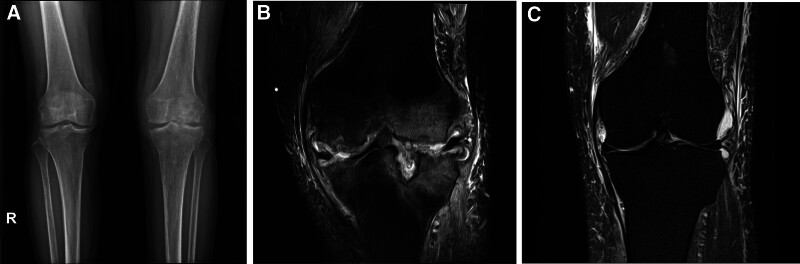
Radiography and magnetic resonance imaging revealed notable erosive and osteolytic changes around the joint surface (A and B), which had progressed rapidly since 3 mo prior (C). R = resistant.
